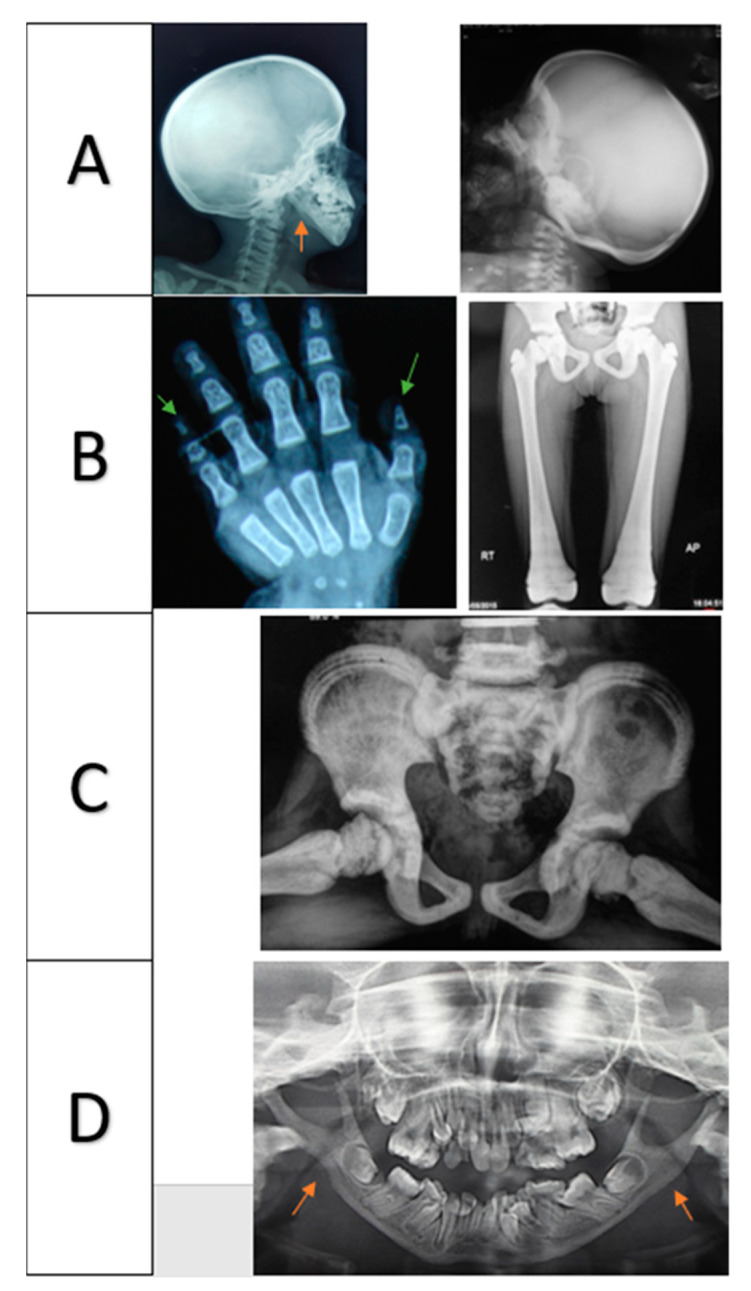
(A) Skull showing thick calvarium, open anterior fontanelle, and wormian bones; red arrows indicate straight mandibular angles. (B,C) Increased bone density; green arrows indicate acro-osteolysis of terminal phalanges. (D) Panorama X-rays showing crowding of the teeth; red arrows indicate straight mandibular angles.
